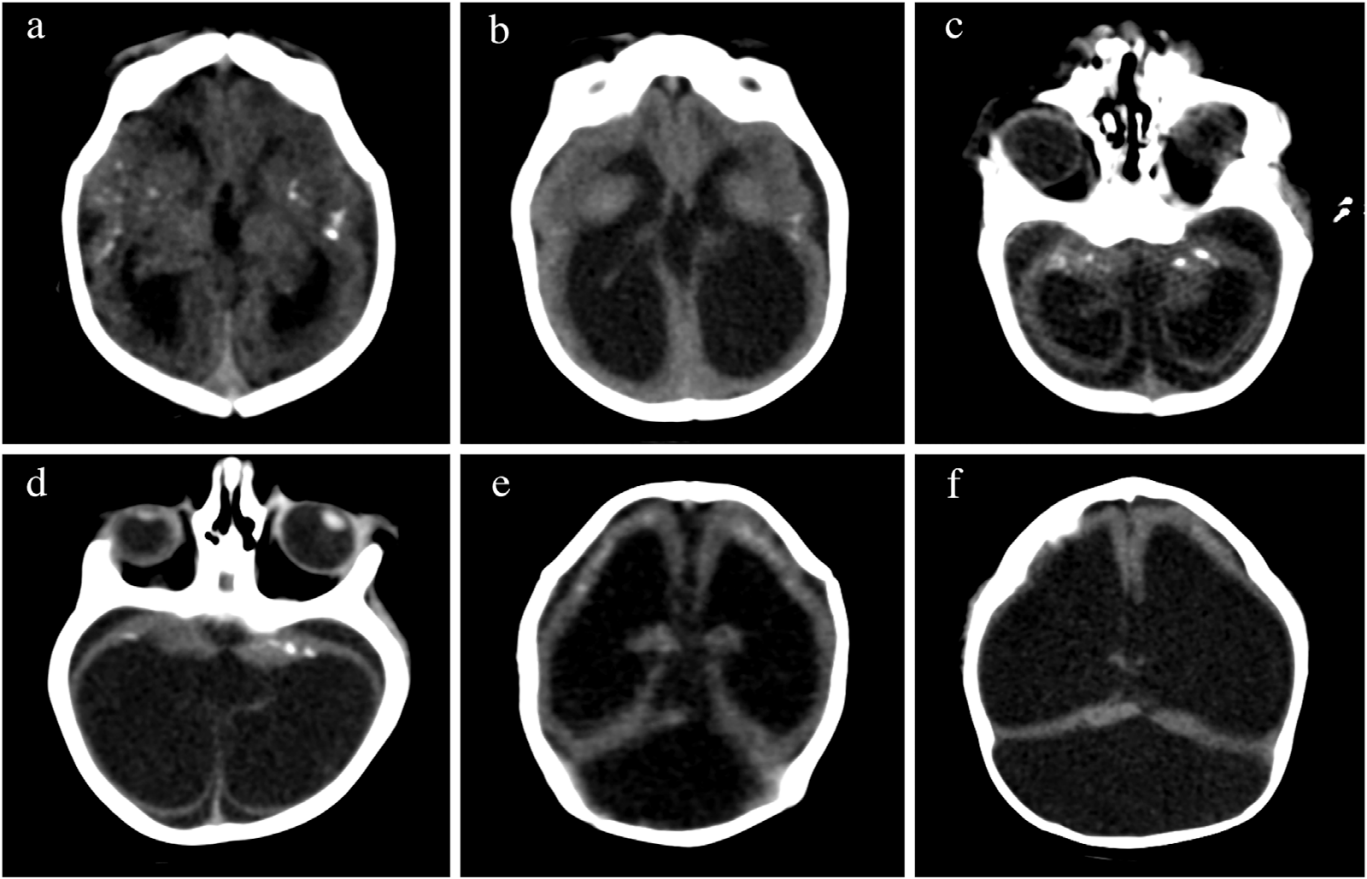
Changes in brain imaging observed during the first year of life. CT of the brain of the same patient in the first month of life in the upper row in (A,C,E), at 6 months of age in lower rows (B,D), and at 13 months of age in (F). Calcifications are more difficult to visualize at 6 months of age (B,D) than at birth (A,B). We observed increases in volume of intraventricular and extra-axial fluids (bottom pictures) in comparison to the initial exam (top pictures). The increasing ventricular dilatation did not show clinical or imaging signs of increased intracranial pressure at 6 months (B,D), but a high tentorium confirmed progressive hydrocephalus requiring surgical shunting at 13 months (F).
